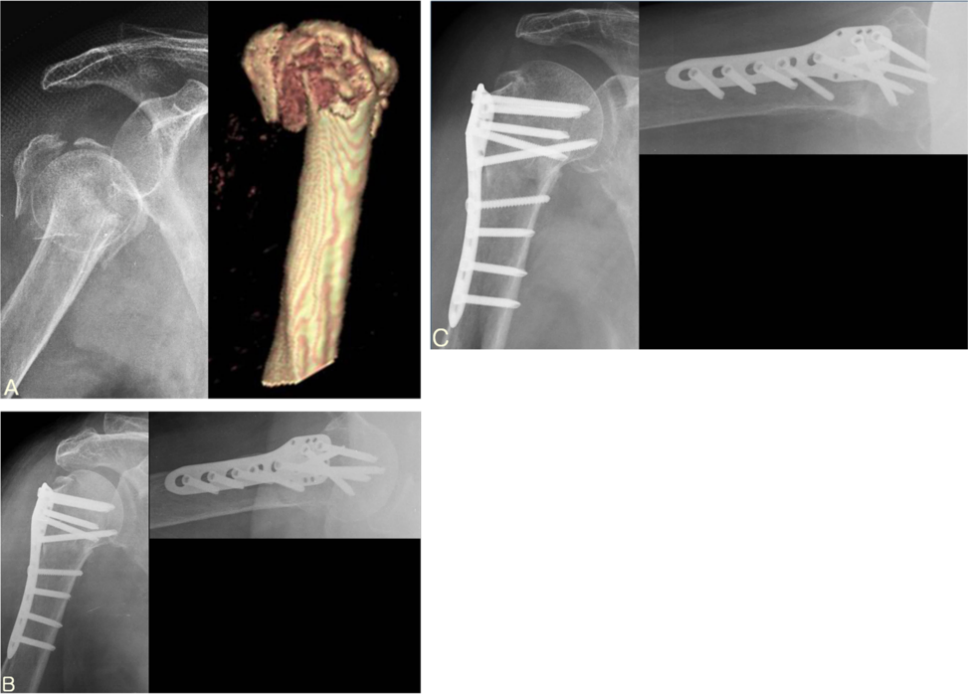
(A) Initial radiograph of 82 years old female with osteoporosis showed 3 part proximal humerus fracture. (B) Immediate postoperative radiograph showed good alignment and successful medial support was achieved by oblique long locking screw. (C) At 12 months follow up, radiograph showed complete bone union and good alignment and the clinical result was excellent.
